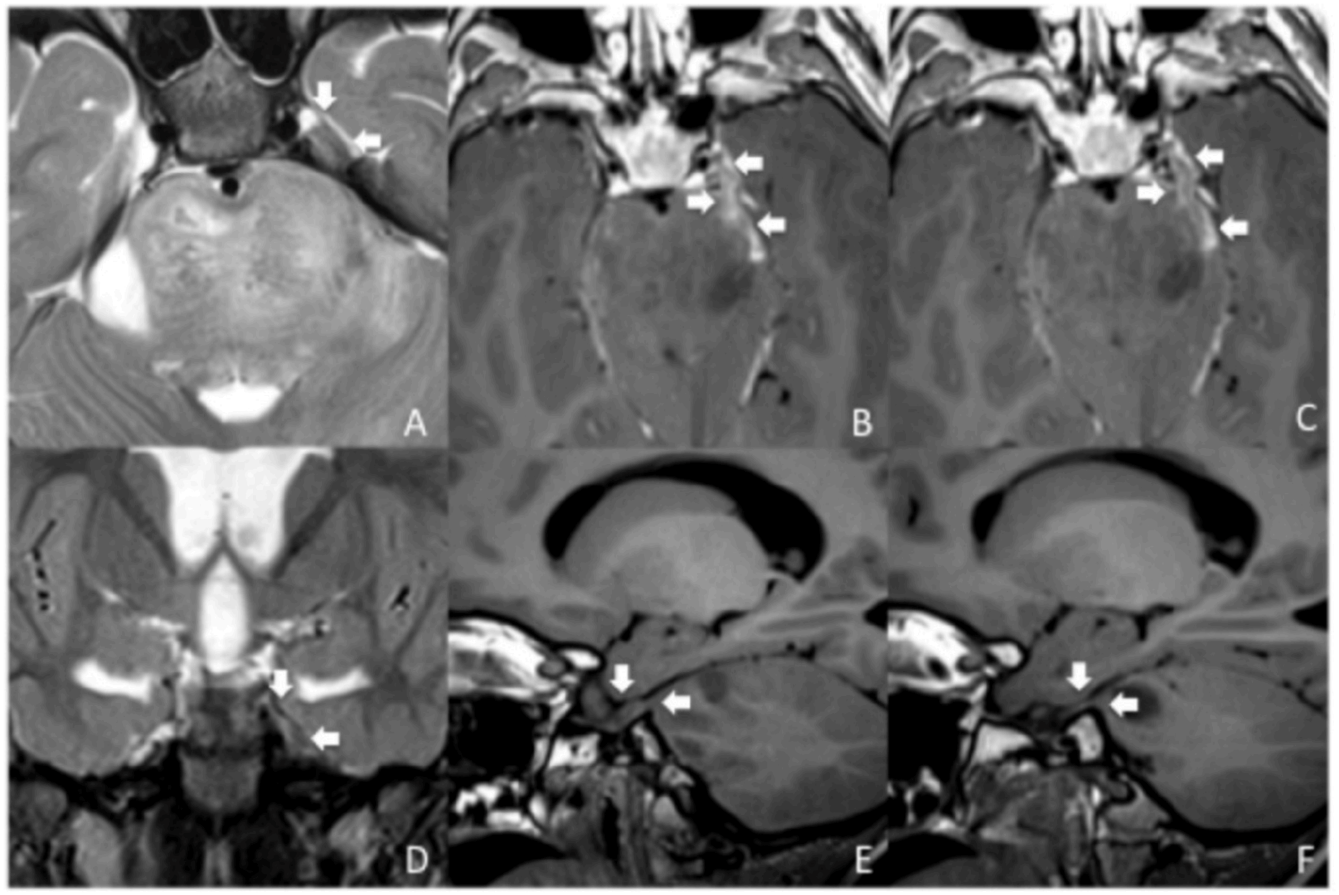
MRI diagnosis of direct involvement of CN V by DIPG in a 4-years-old boy. Axial TSE T2 (A), para-axial reformatted T1 post-contrast (B,C), coronal TSE T2 (D) and para-sagittal reformatted T1 images (E,F) show thickening of the left CN V causing filling of the left Meckel cave, consistent with direct cranial nerve involvement from the tumor (A–E, white arrows). The pathologic thickening of the left CN V is associated with contrast enhancement (B,C, white arrows). Pathologic thickening of CN V is better delineated when comparing it with the contralateral normal V cranial nerve (F, arrows).
